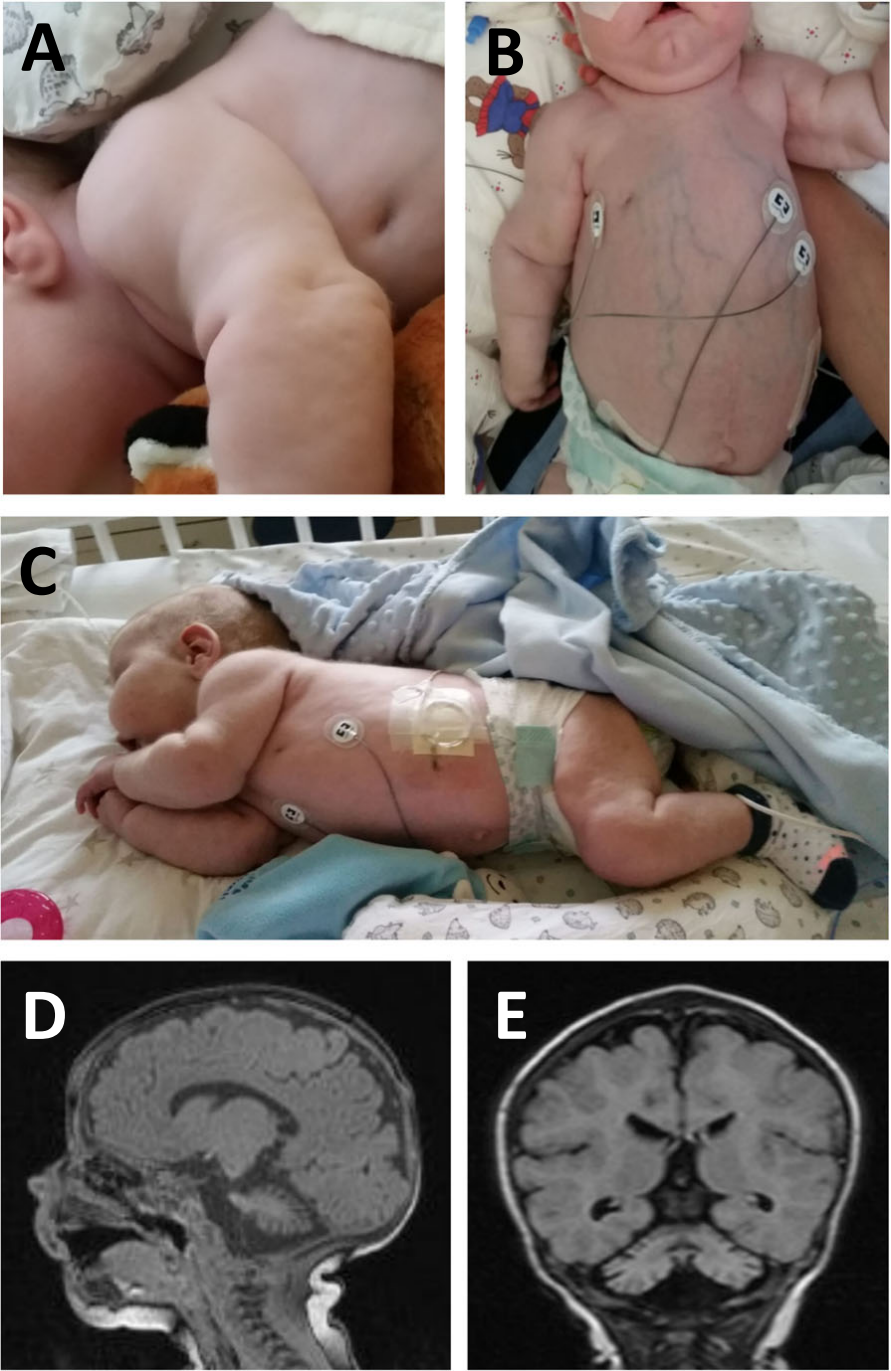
Characteristic clinical findings of PMM2-CDG. a-c Inverted nipples, abnormal subcutaneous fat distribution, ascites. d-e Brain MRI of the patient at age 2.5 months showing cerebellar atrophy
